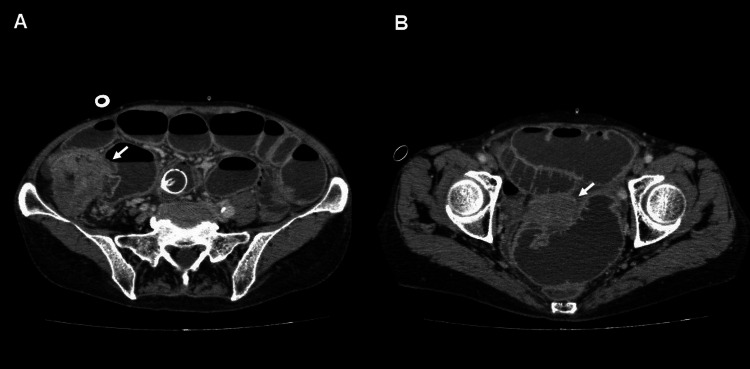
Preoperative CT findings. (A) Ileocecal cancer measuring 7 cm in diameter (arrow), causing intestinal obstruction. An ileus tube was placed.
